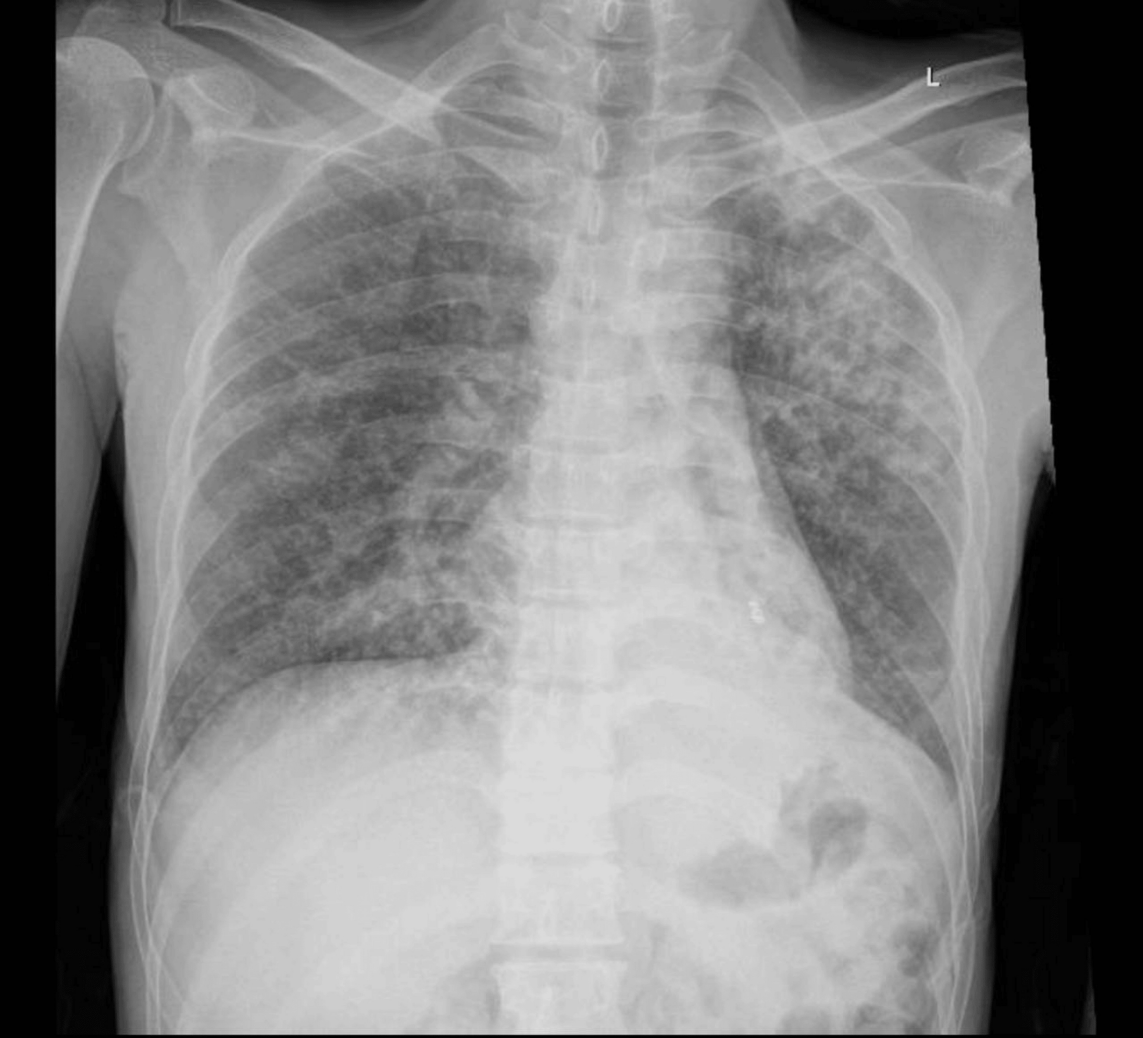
Chest x-ray demonstrated dense left upper-zone consolidation with a subclavicular cavity and marked interval improvement compared with previous radiographs.
